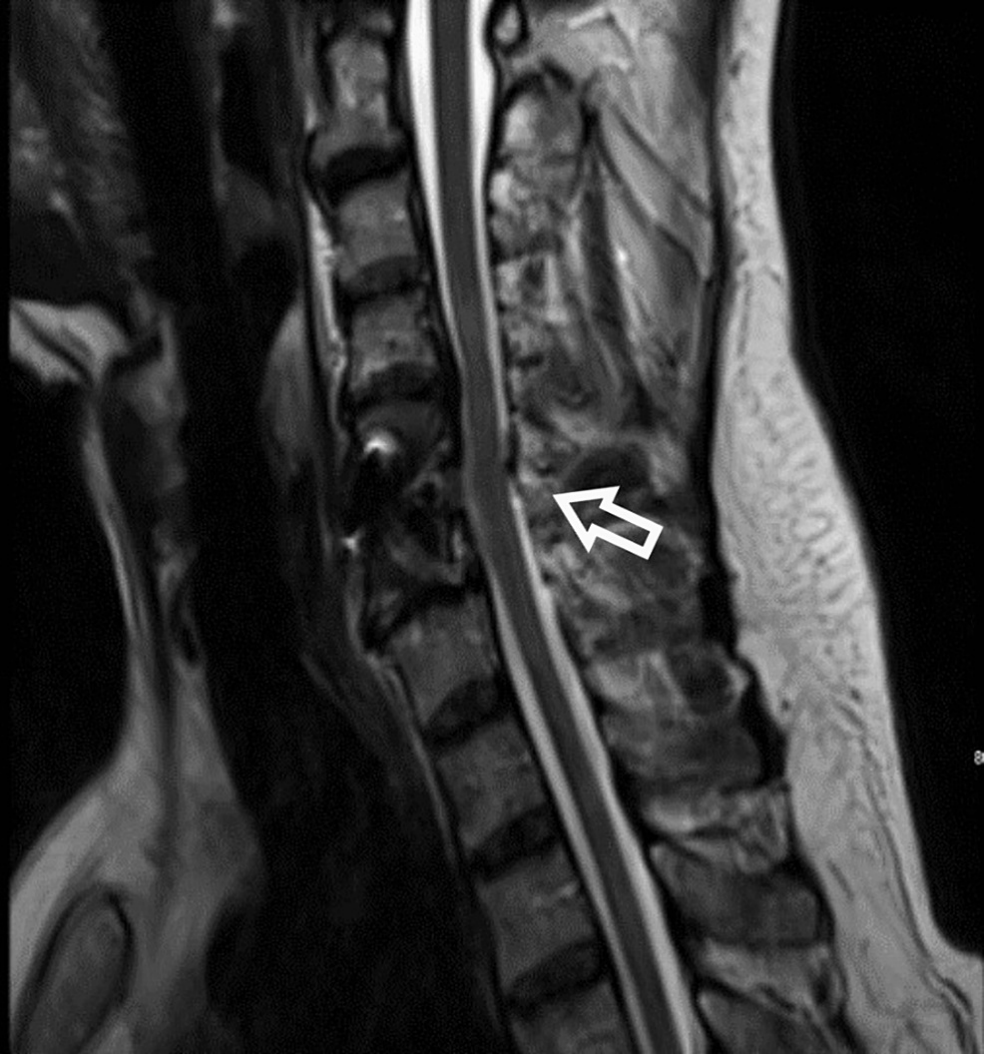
Magnetic resonance imaging sagittal view post anterior cervical discectomy and fusion at level C5/6. Immediate post-op magnetic resonance imaging scan showed no compressing pathology and good spinal decompression however there is evidence of spinal cord expansion and swelling.
